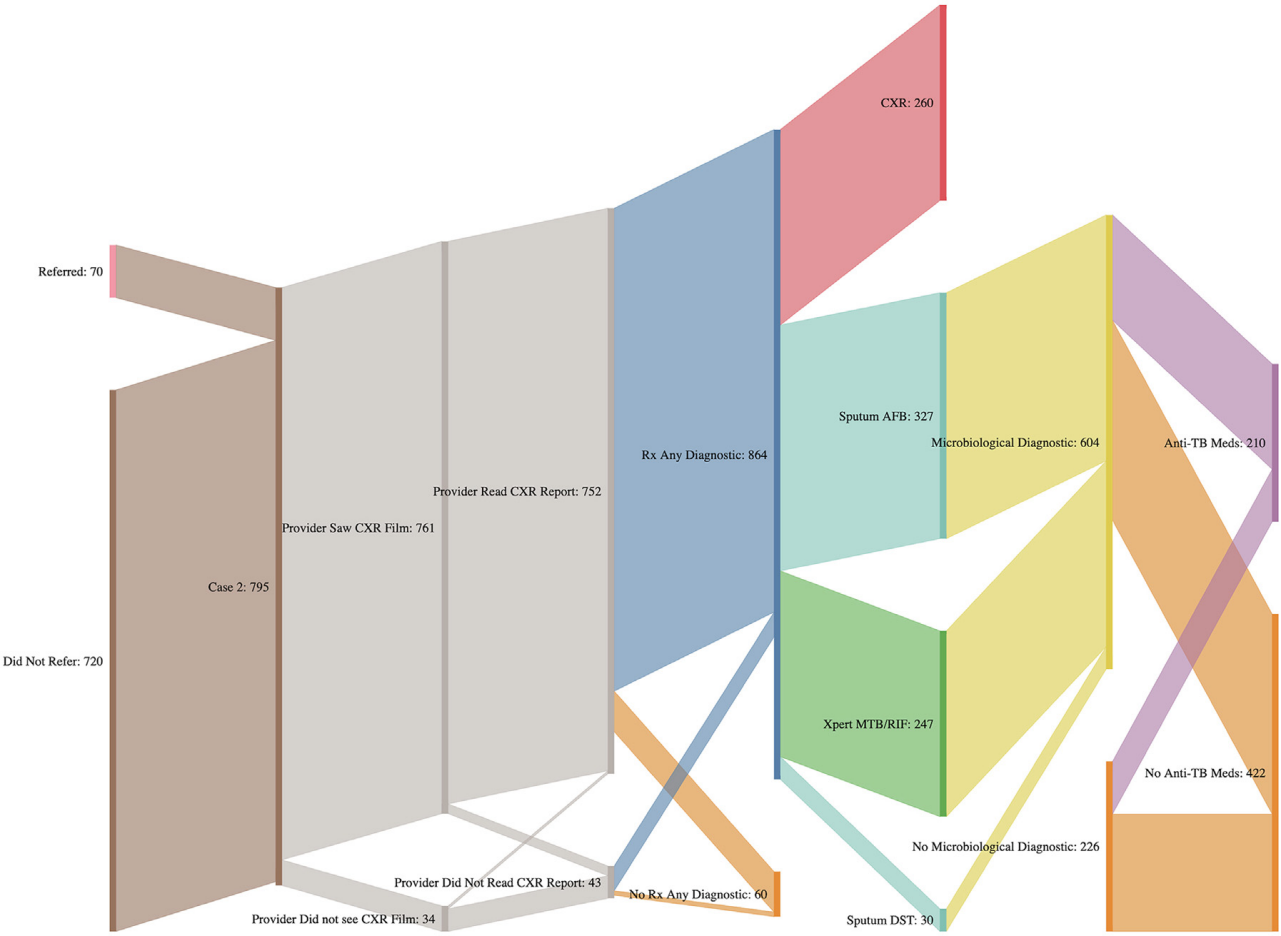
Diagnostic care cascade of patients presenting with an abnormal CXR (Case 2) and the subsequent diagnostic algorithm by which they travel through, with the dispensation (or not) of ATT regimens they are dispensed as the endpoint. These represent raw counts. CXR = chest radiography; DST = drug-susceptibility testing; AFB = acid-fast bacillus.
